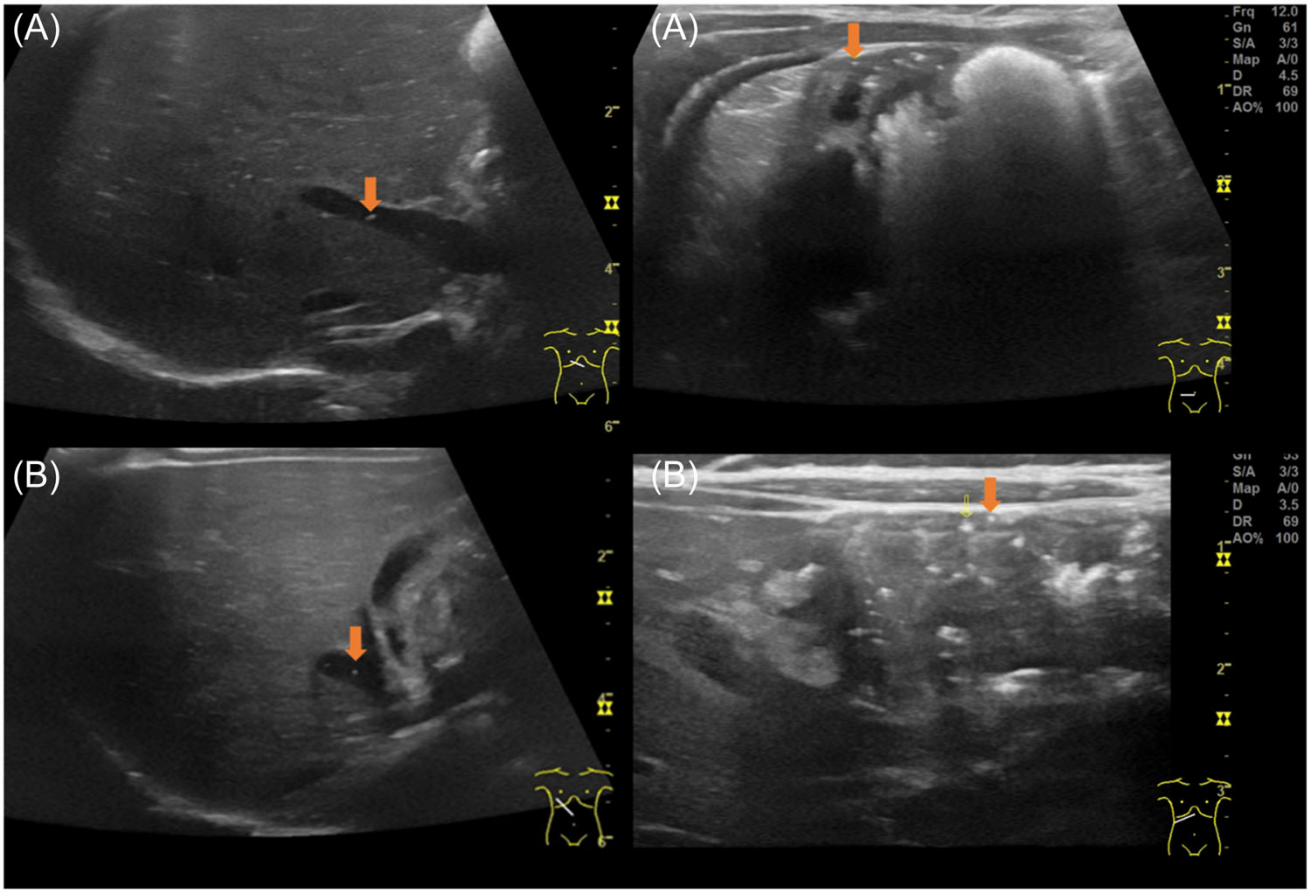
Ultrasound findings of aeroportia (left side) and pneumatosis intestinalis (right side) in patient A and patient B.
